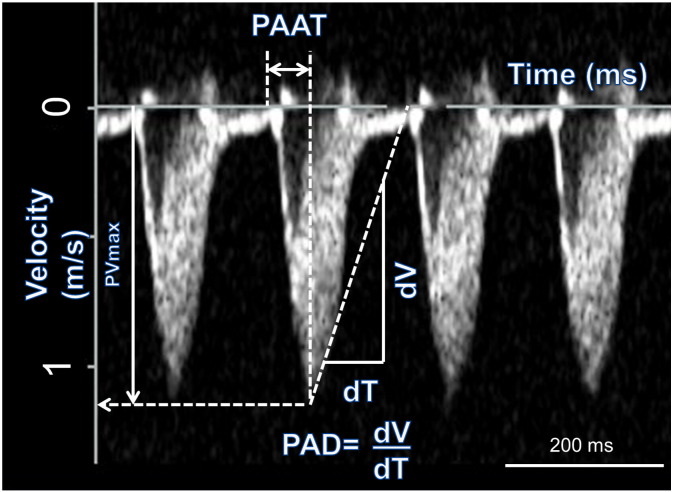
Pulsed wave Doppler recording through the pulmonary artery and measurement of PVmax, PAAT and PAD. The x axis measures time and the y axis measures velocity. PAAT is the time from the beginning of flow to the peak velocity, measured from the x axis. PVmax is the maximum velocity measured from the y-axis. PAD is the gradient of the initial deceleration of the pulmonary velocity profile.
